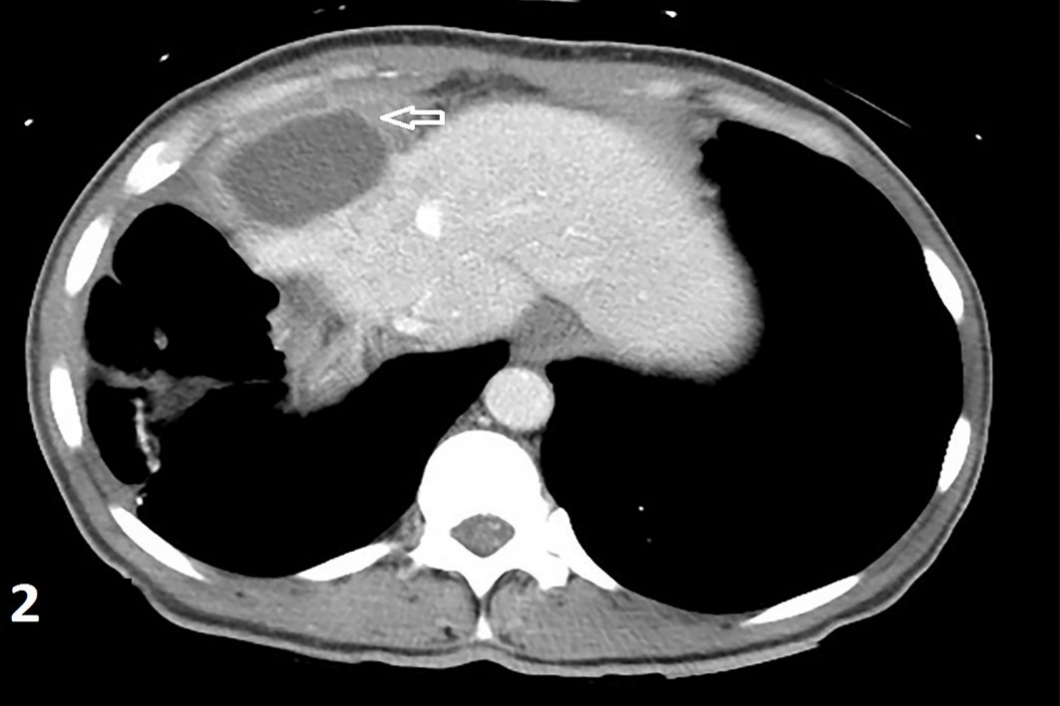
Computed tomography scan (abdomen) showed a dominant 5.2x5.5x3.9 cm hypodense lesion consistent with an abscess above the right liver
